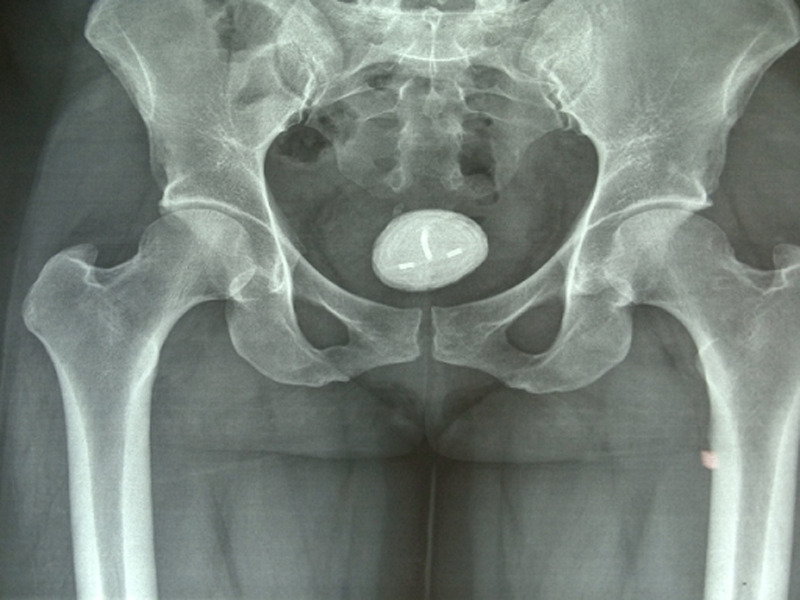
plain X-ray of the pelvis; a calcified bladder mass is noted with the intrauterine contraceptive device embedded in it
